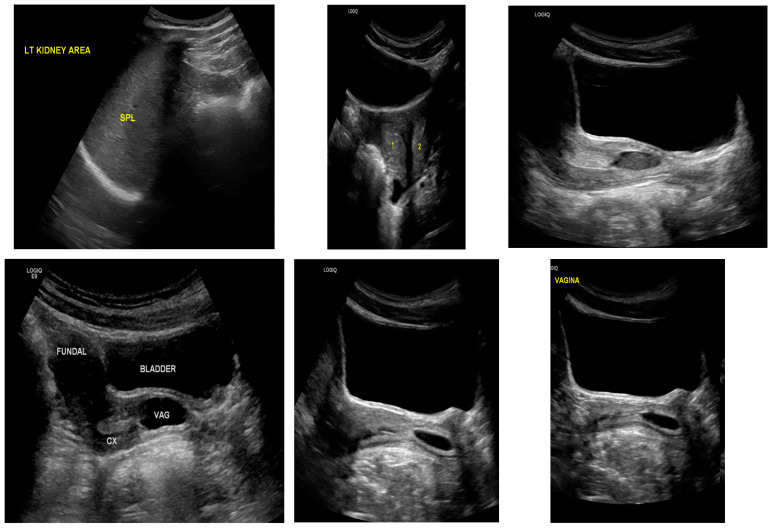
Transabdominal pelvic ultrasound in an 11-year-old prepubertal patient (Case 4) demonstrating cystic structures along the left vaginal wall, without evidence of hematocolpos at the time of examination.
